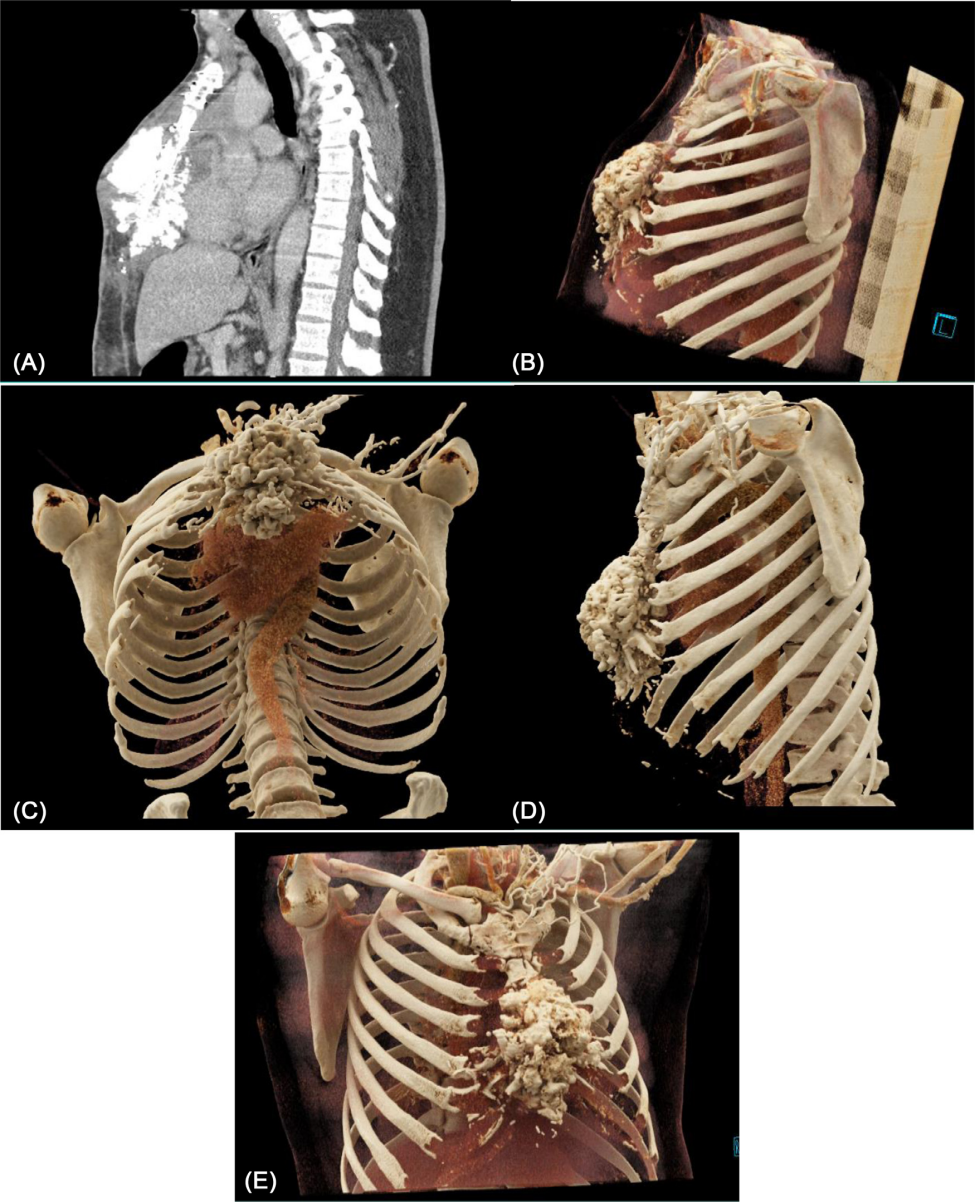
A) Sagittal CT scan demonstrates osteoid matrix involving chest wall, sternum, and retrosternal space, (B–E) Cinematic renderings define the extent of tumor matrix and involvement of sternum and chest wall.
